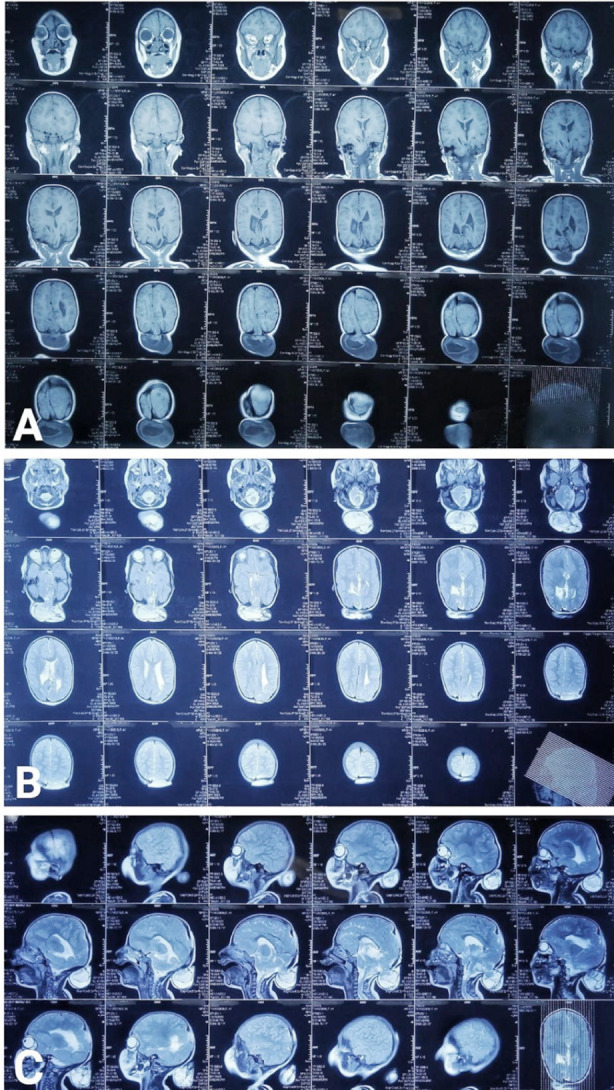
A: MRI brain axial T1WI showing occipital and suboccipital encephaloceles, B: Axial T2WI showing both the encephaloceles, C: Sagittal T2WI showing small occipital and large suboccipital encephalocele.
